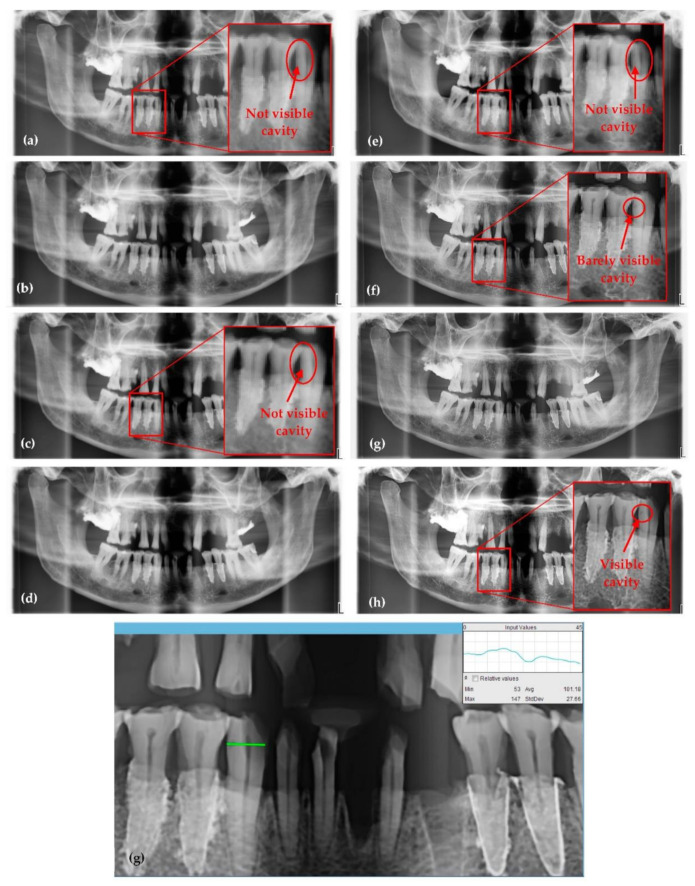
(a–h) Panoramic radiographs obtained during the optimization process. (g) Area with detail (small cavity) followed on each radiography image using OCT images as reference. The settings of the X-ray unit to perform each image and the quality parameters around the small cavity are provided in Table 1. The quality parameters of the entire radiographic images are compared in Table 2.
